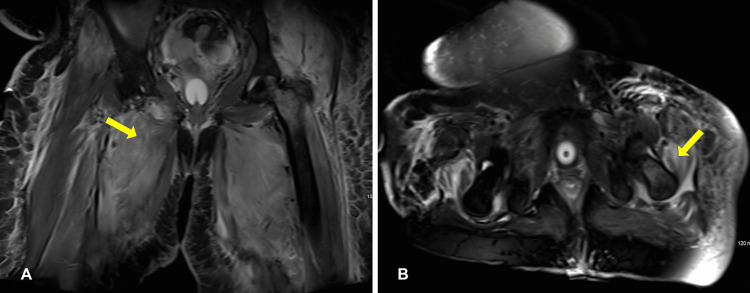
Magnetic Resonance Image of her Thigh and Femur Generally showed diffuse subcutaneous edema bilaterally, Some left femoral bone marrow edema in the neck and trochanteric region could represent transient osteoporosis versus bone contusion. No cortical bone destruction. (A) Coronal view of pelvic T1 weighted MRI with increased signal intensity of adductor longus muscle (arrow). (B) Axial view of pelvic T1 weighted MRI with increased signal intensity of gluteus medius tendon (arrow). All findings were suggestive of myositis. Abbreviation: MRI, magnetic resonance imaging.
